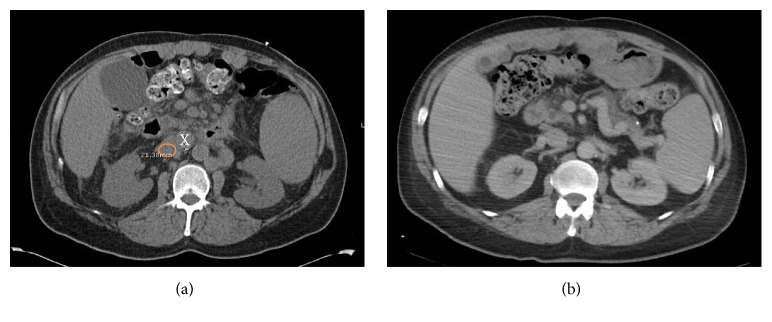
(a) CT without contrast. Pretreatment lymph node (X) is 2.1 cm. Orange circle is IVC. (b) CT with contrast. Posttreatment lymph node on a comparable level is not visible.
